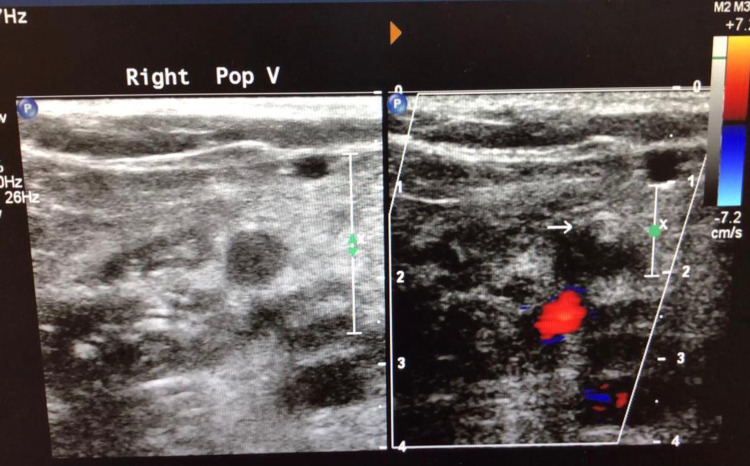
Colour Doppler ultrasound scan image showing thrombus in right popliteal vein
